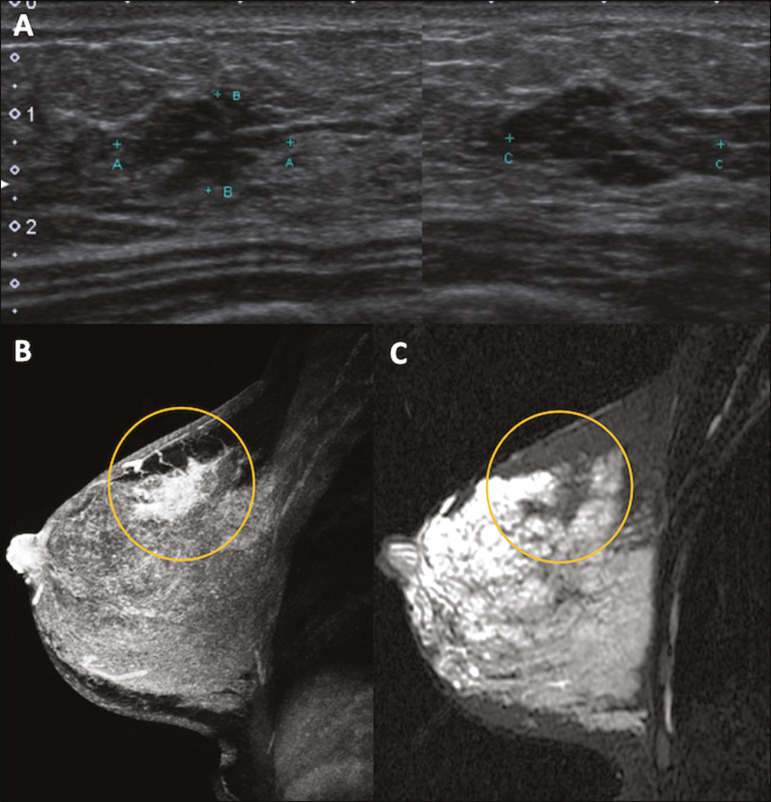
A 38-year-old patient in the sixth month of puerperium. Ultrasound (A) showed an irregular, hypoechoic mass with microlobulated margins. Postcontrast T1 (B) and T2 (C) weighted MRI images showed a non-mass enhancement in the upper outer quadrant of the right breast, with low signal in T2, unlike the rest of the breast parenchyma, which shows intermediate to high signal due to the lactation state.
